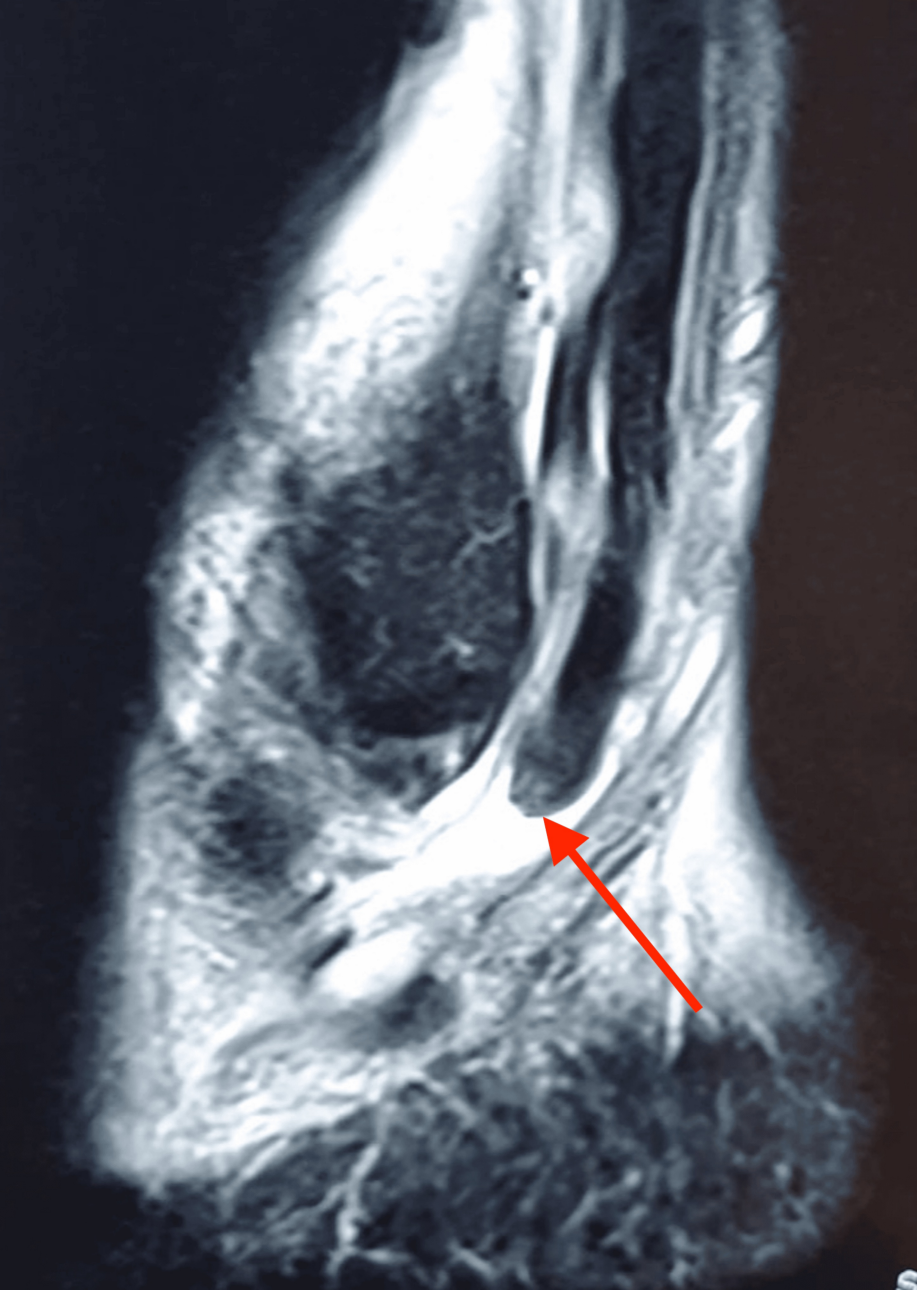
MRI sagittal image of the patient showing the proximal stumps (red arrow) of the ruptured peroneal brevis and longus tendons.
